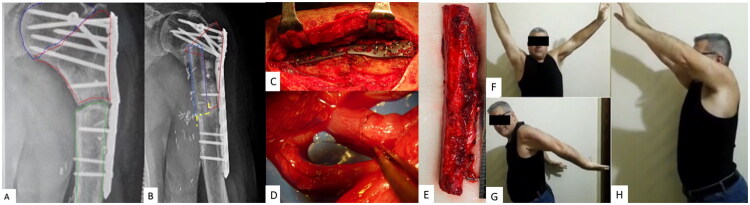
(Case 1). A. X-ray after first surgery showing oncological resection, reconstruction with intercalary allograft and broken plate. Humeral head is surrounded by blue dashed line. Intercalary allograft is surrounded by red dashed line. Humeral shaft is surrounded by green dashed line. B. X-ray showing radiological healing of the hybrid procedure at 10 months after second surgery. Intercalary allograft is surrounded by red line. FVFG is surrounded by blue dashed line. Step-cut osteotomy is underlined by yellow dashed line. C. Intraoperative picture of the broken plate. D, E. Microvascular anastomosis and FVFG. F, G, H. Clinical pictures of the patient with satisfactory range of motion of the left arm at 12 months after surgery.
